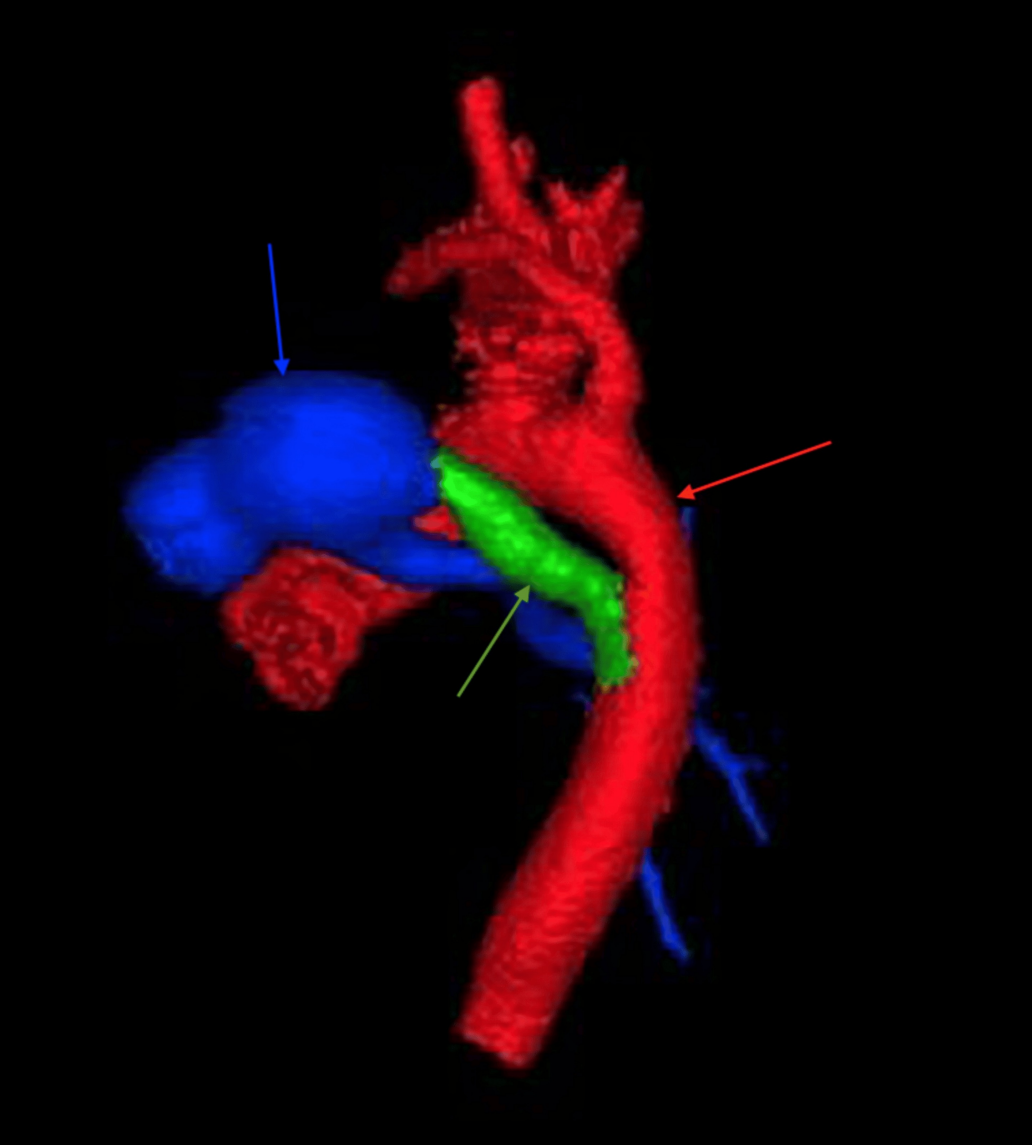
Three-dimensional computed tomography angiography reconstruction demonstrating patent ductus arteriosus (green arrow) connecting pulmonary artery (blue arrow) and aorta (red arrow)
